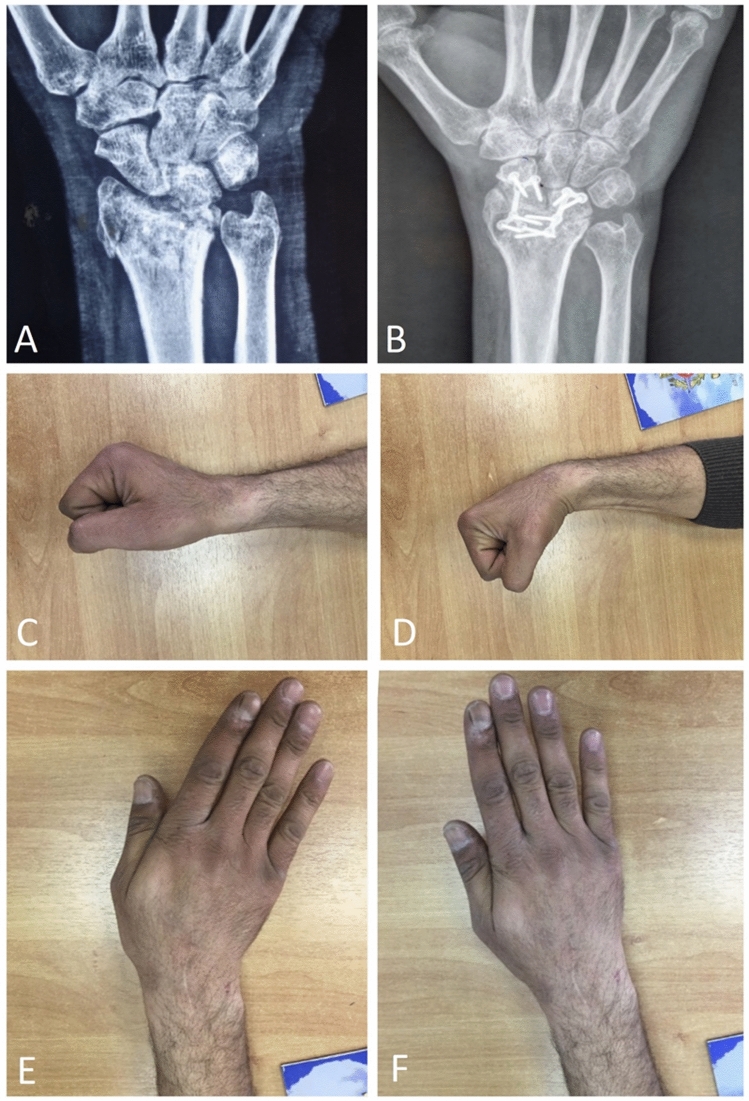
A Case example of RSL fusion after intra-articular distal radius malunion, A preoperative posteroanterior wrist radiograph, B final posteroanterior radiograph after union of RSL arthrodesis, C final wrist extension range, D final wrist flexion range, E: final ulnar deviation range, F final radial deviation range (limited)
